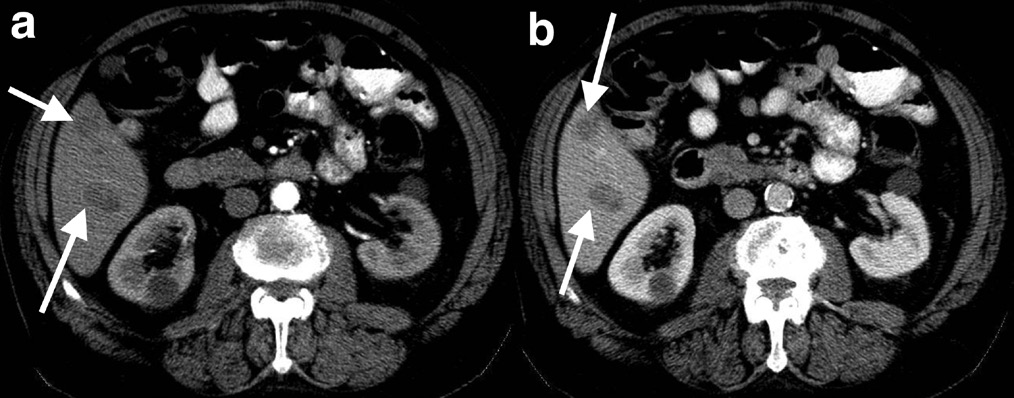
Hypovascular GIST liver metastases. Multiphase CT images demonstrates segment 5 GIST liver metastases are hypoattenuating (arrows) in the arterial phase (a) and portal venous phase (b). GIST, gastrointestinal stromal tumour.
